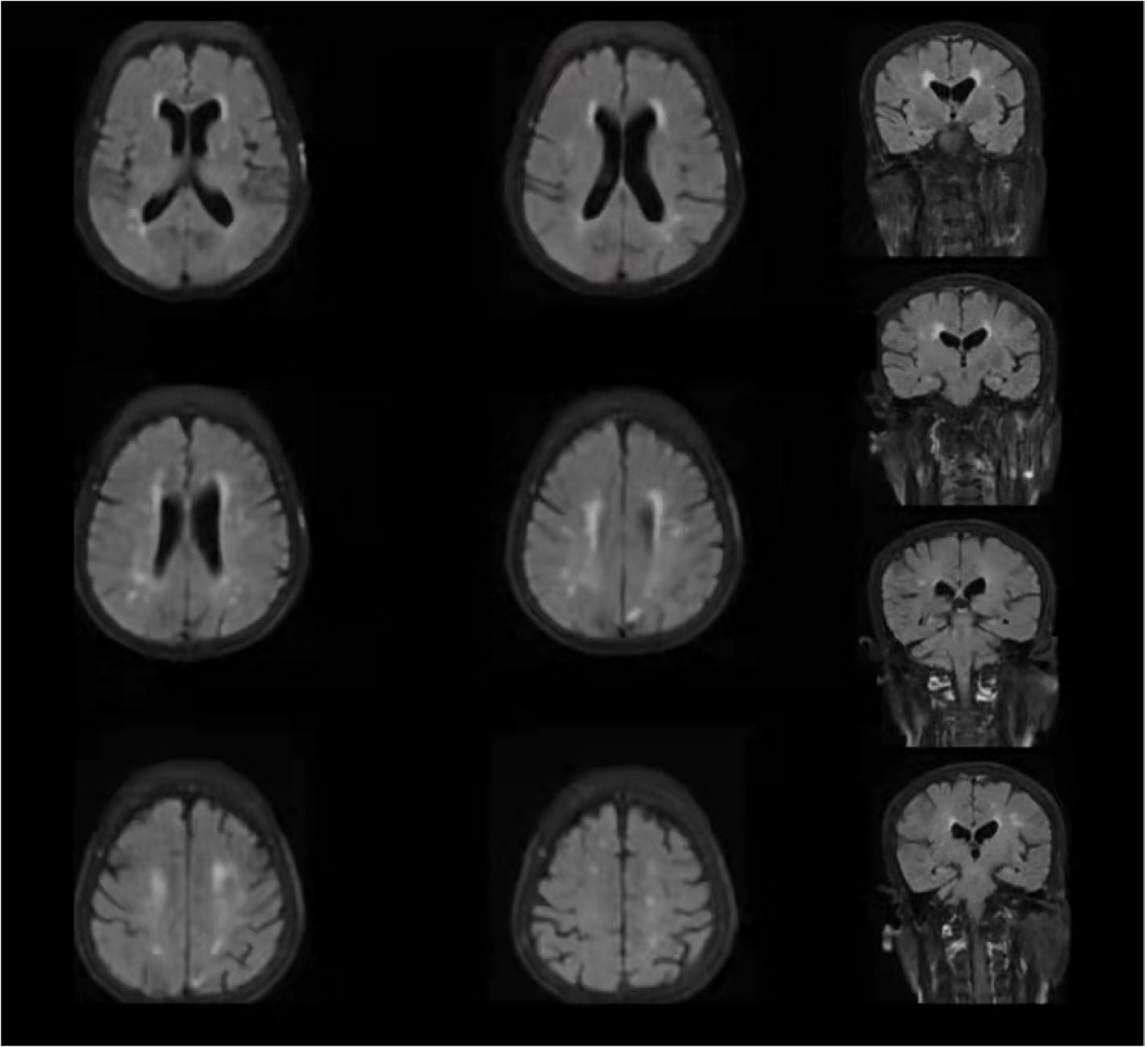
The patient’s MRI results
